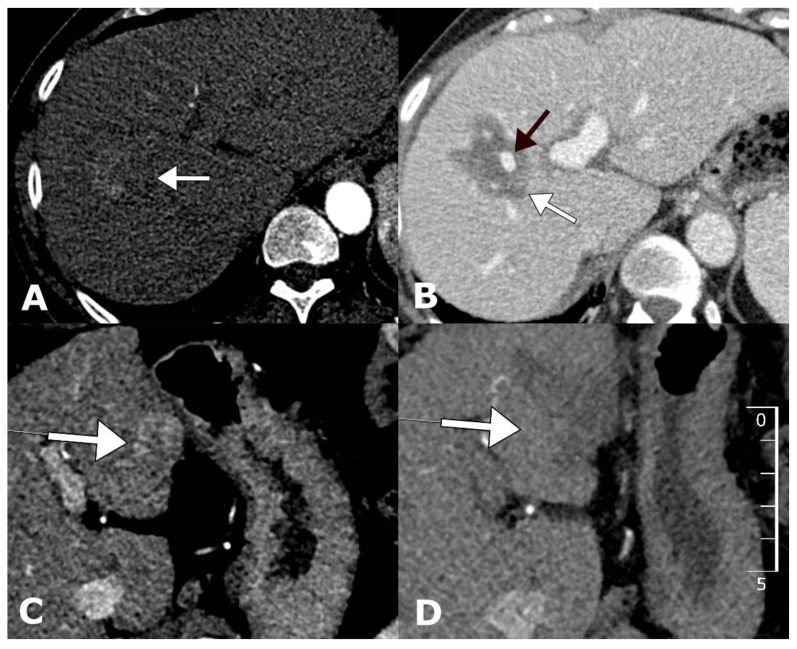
Examples of tumors located centrally and peripherally before and after treatment with ECT. Arterial-phase contrast-enhanced computed tomography (CECT) demonstrates centrally located HCC with a diameter of 31 mm located in the vicinity of a major portal vein branch (A). Follow-up CECT 1 month after ECT demonstrates a complete response with no tumor enhancement and a patent portal vein branch (B). Arterial-phase CECT demonstrates peripherally located HCC in liver segment III (C). Follow-up CECT 1 month after ECT demonstrates a complete response with no tumor enhancement (D).
